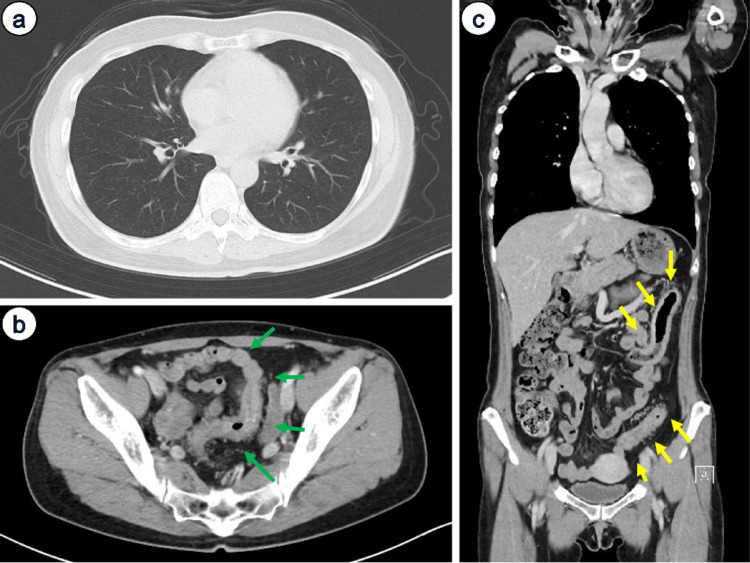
Computed tomography (CT) findings after upadacitinib initiation. a–c Contrast-enhanced CT revealed wall thickening of the left colon, with no abnormal findings in other organs (a axial image at the level of the lungs, b axial image at the level of the sigmoid colon, and c coronal image). Green arrows indicate the sigmoid colon; yellow arrows indicate the left transverse and sigmoid colon
